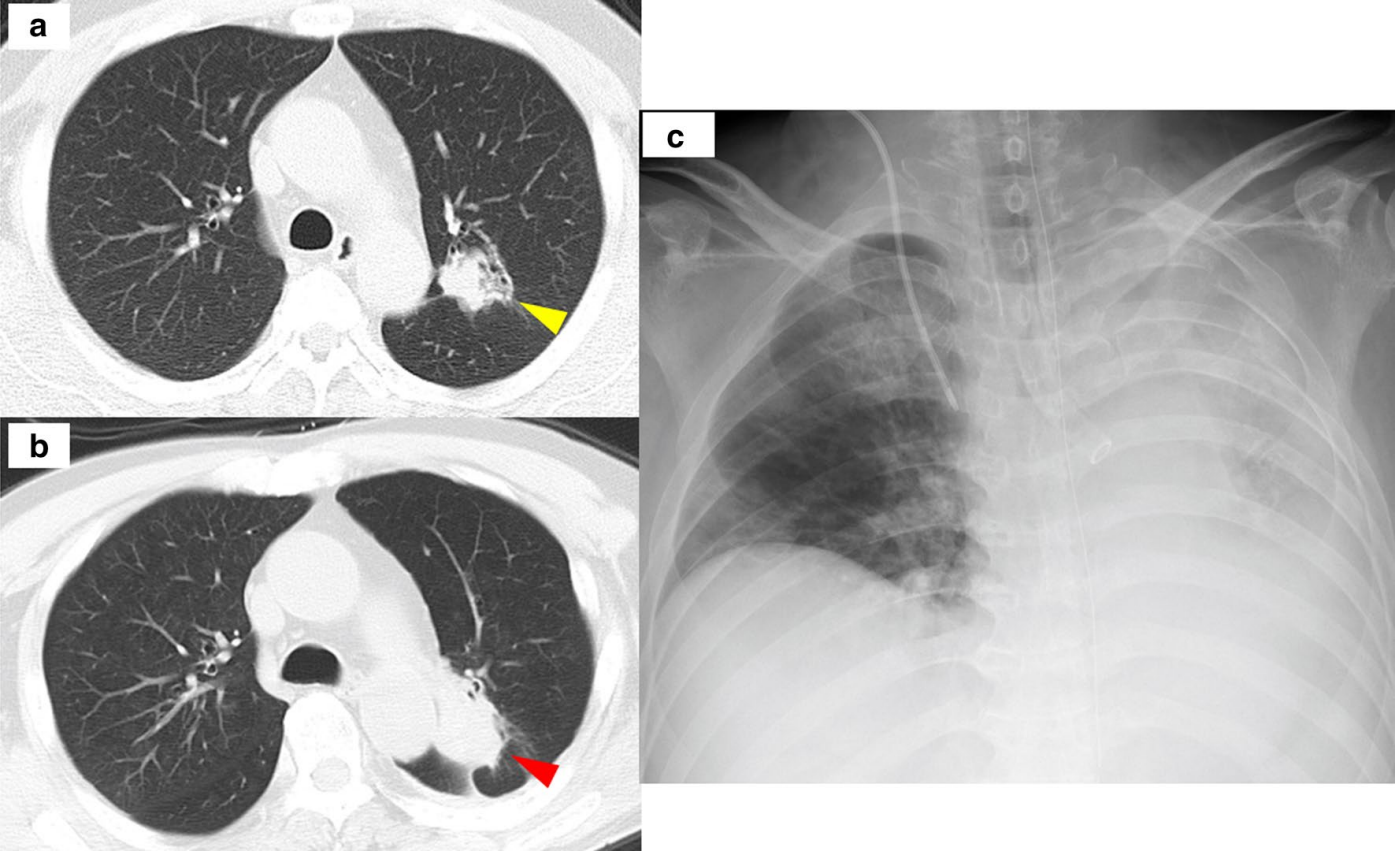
Preoperative imaging. a Computed tomography before proton bean therapy (yellow arrowhead). b Computed tomography of lung cancer recurrence (red arrowhead). c Chest X-ray after massive hemoptysis. *PBT* proton beam therapy
